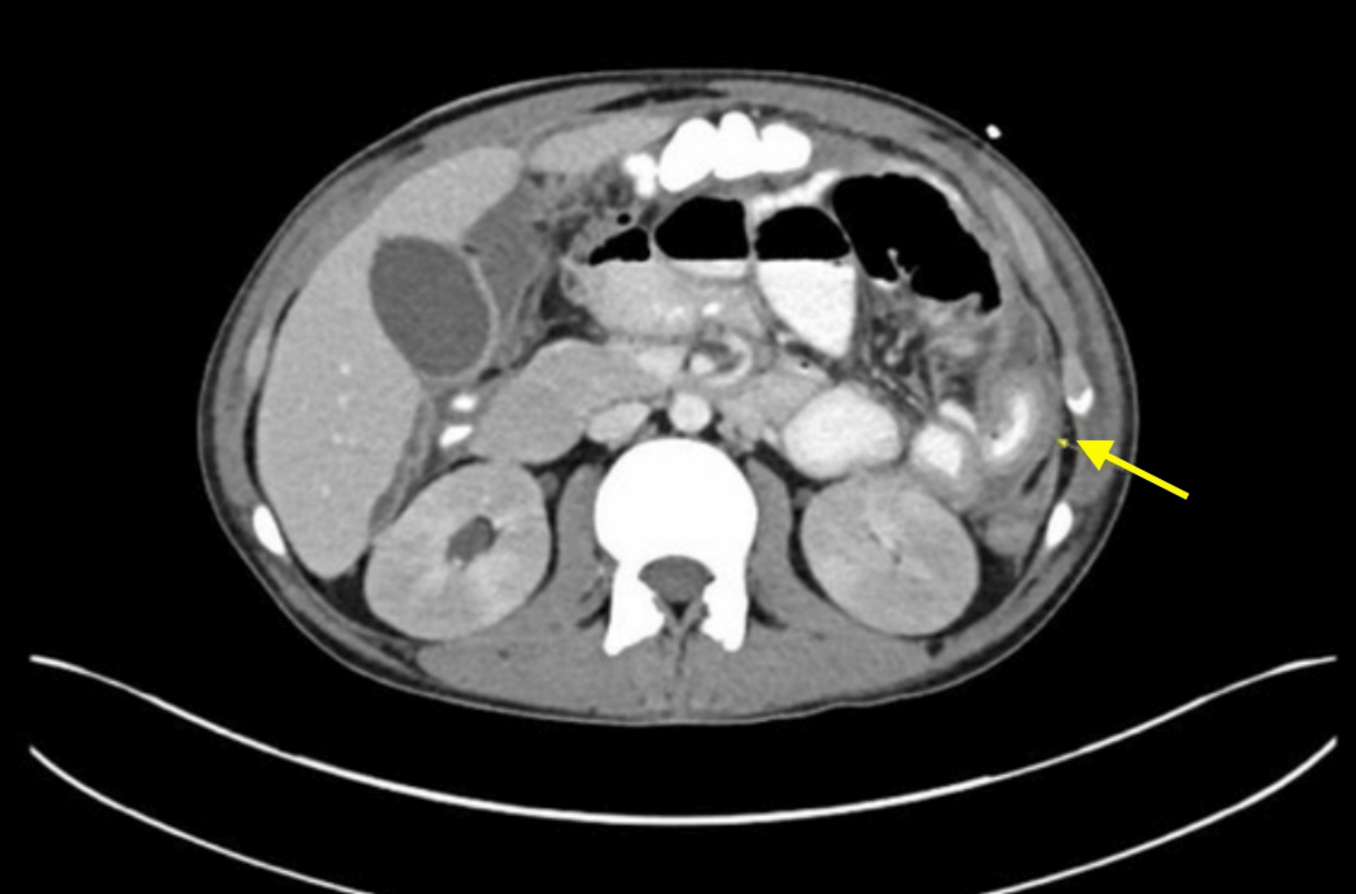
CT abdomen and pelvis with oral contrast showing new circumferential wall thickening and mucosal hyperenhancement of a small segment of patulous ileal bowel loop, as well as contrast leak concerning for small bowel leak
